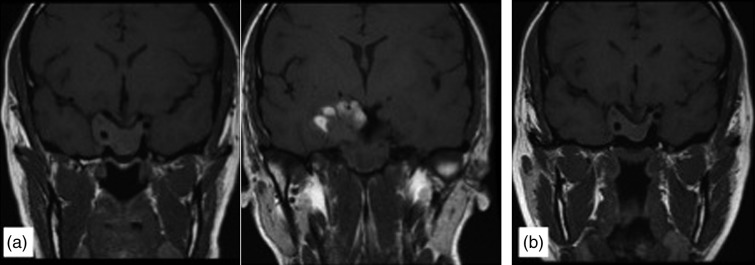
(a) Interval magnetic resonance images (MRI) after 3 months of cabergoline therapy shows dramatic reduction in the size of the tumour with decompression of the midbrain and some new haemorrhagic components. (b) Interval MRI after 10 months of cabergoline therapy shows continued reduction in tumour bulk but the rate of change is slower.
